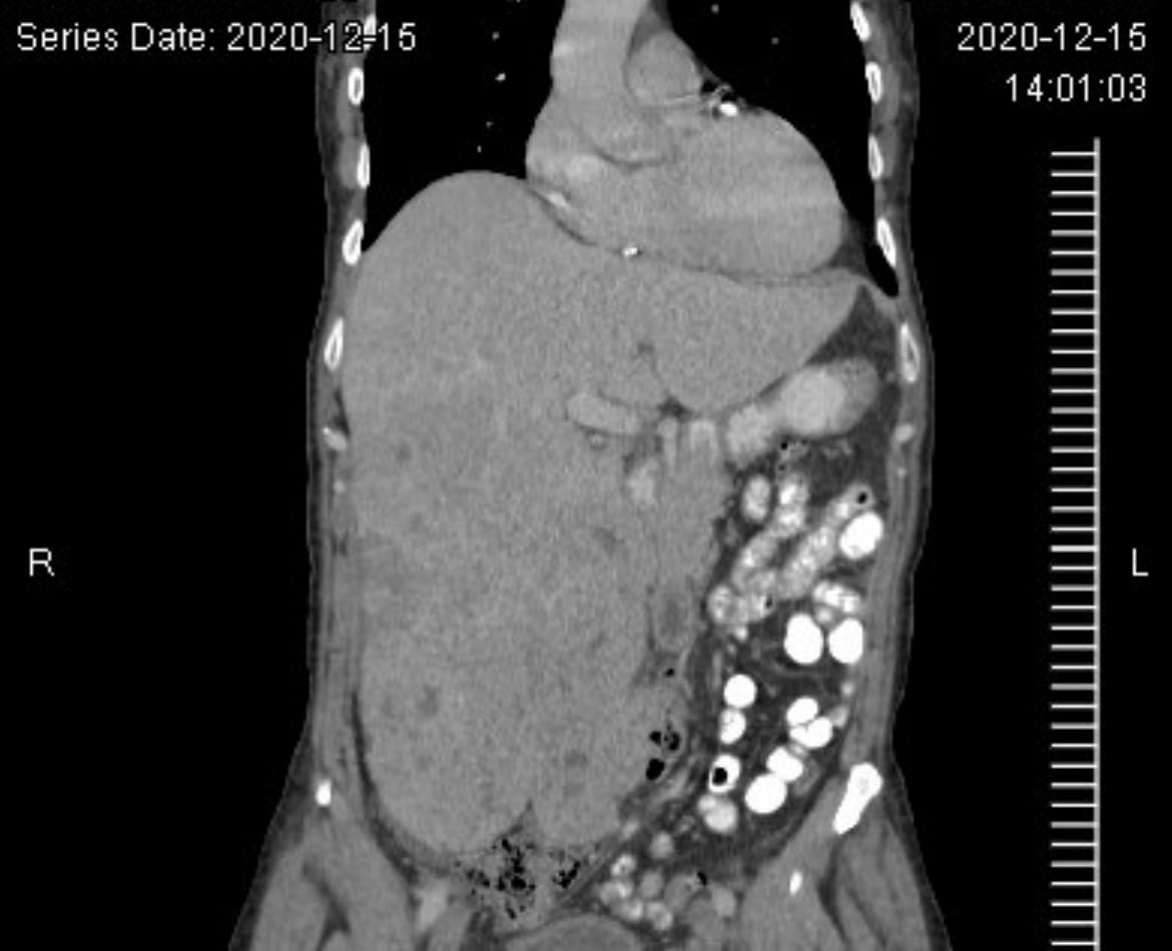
Abdominal CT image. CT image demonstrating massive tumor recurrence in the liver after surgical resection of primary tumor and prior to initiation of temsirolimus.
